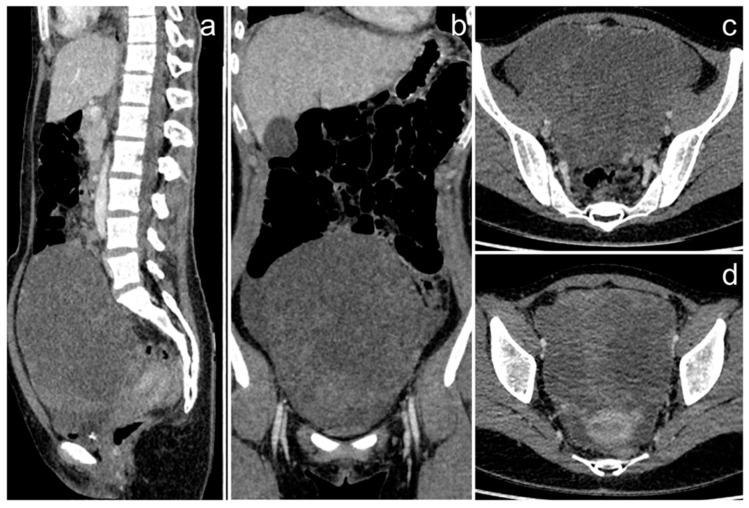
The CT exam of the primary ovarian angiosarcoma (a–d). Extensive, cystic-solid mass in the pelvis (images courtesy of Dr. M. I. Furmanek, Medical University of Warsaw).
